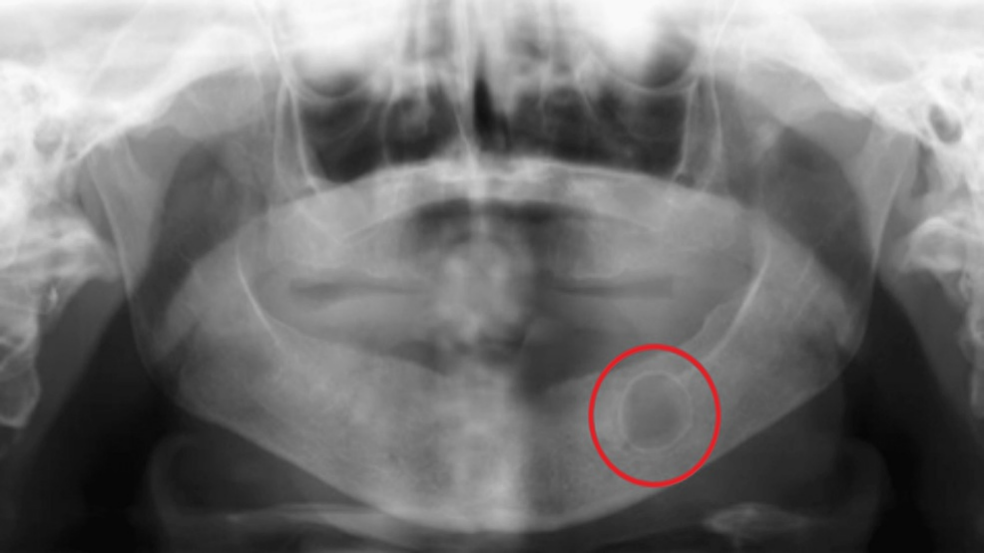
Radiolucent lesion as seen on a panoramic radiograph
